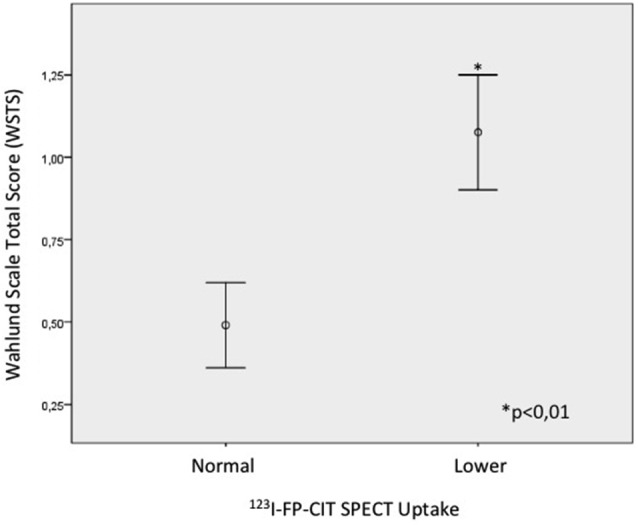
Association between WSTS and abnormal dopaminergic uptake in ^123^I-FP-CIT SPECT (*p* < 0.01).
